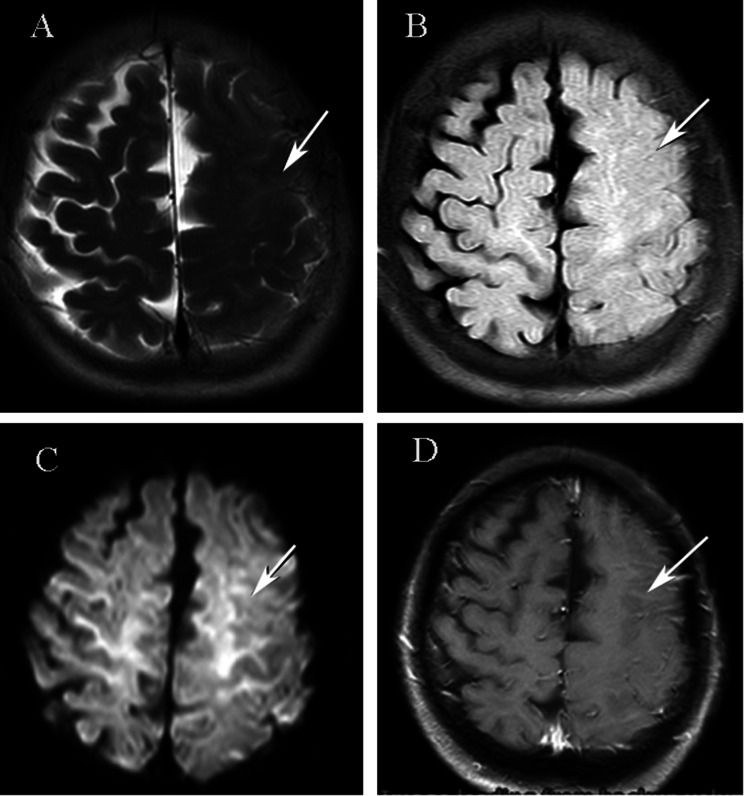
(A) T2-weighted MRI showing gyral swelling with disappearance of sulci over the left frontoparietal lobe. (B). T2-FLAIR demonstrating increased signal with obliteration of sulci due to leptomeningeal thickening over the left frontoparietal lobe. (C). Diffusion-weighted imaging showing an increased signal in the subarachnoid space related to a viscous exudate. (D). Axial post-gadolinium T1-weighted image demonstrating unilateral enhancement of the left leptomeninges
